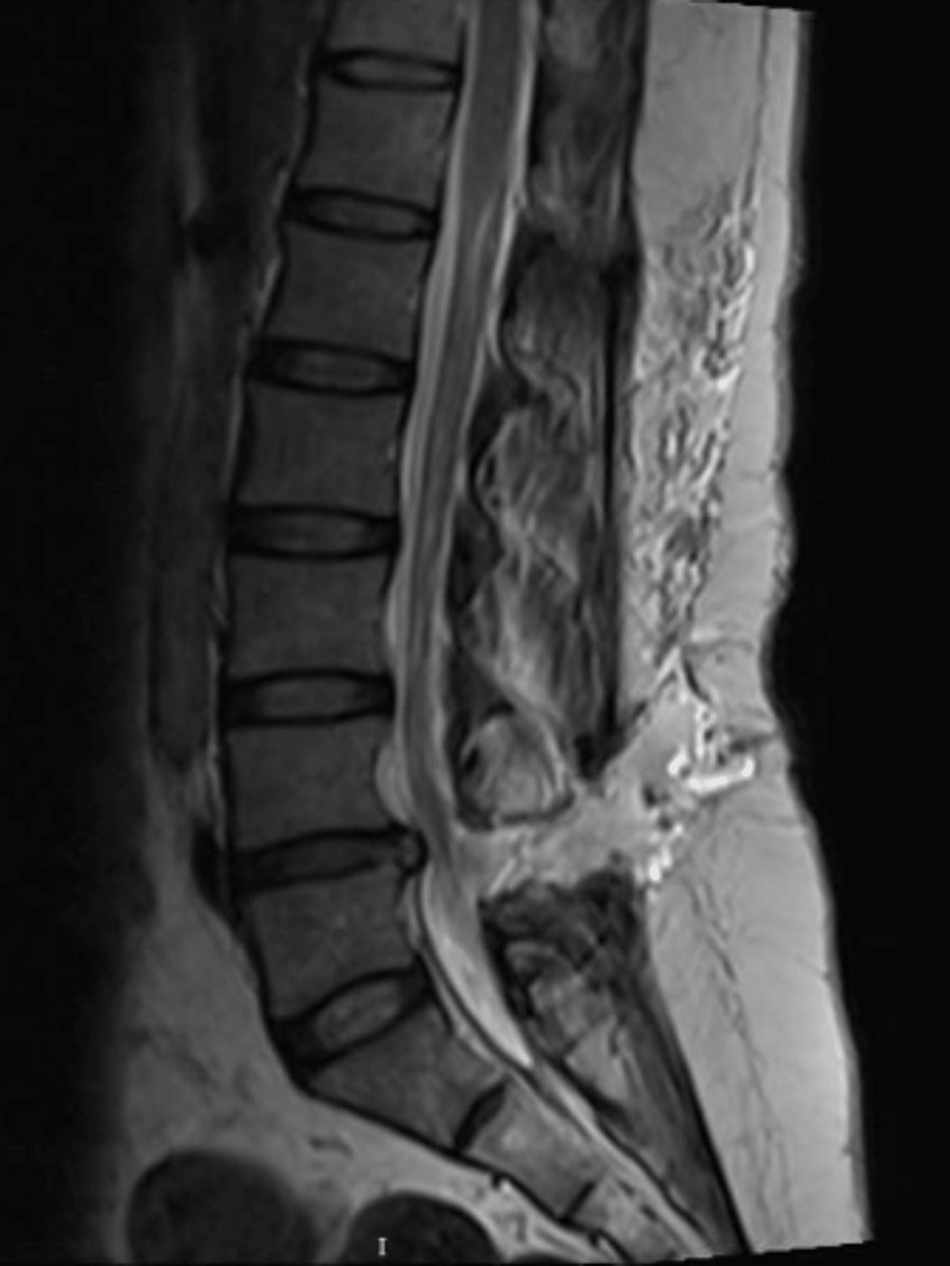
Lumbar spine MRI, sagittal T2, showing a stable epidural CSF collection
